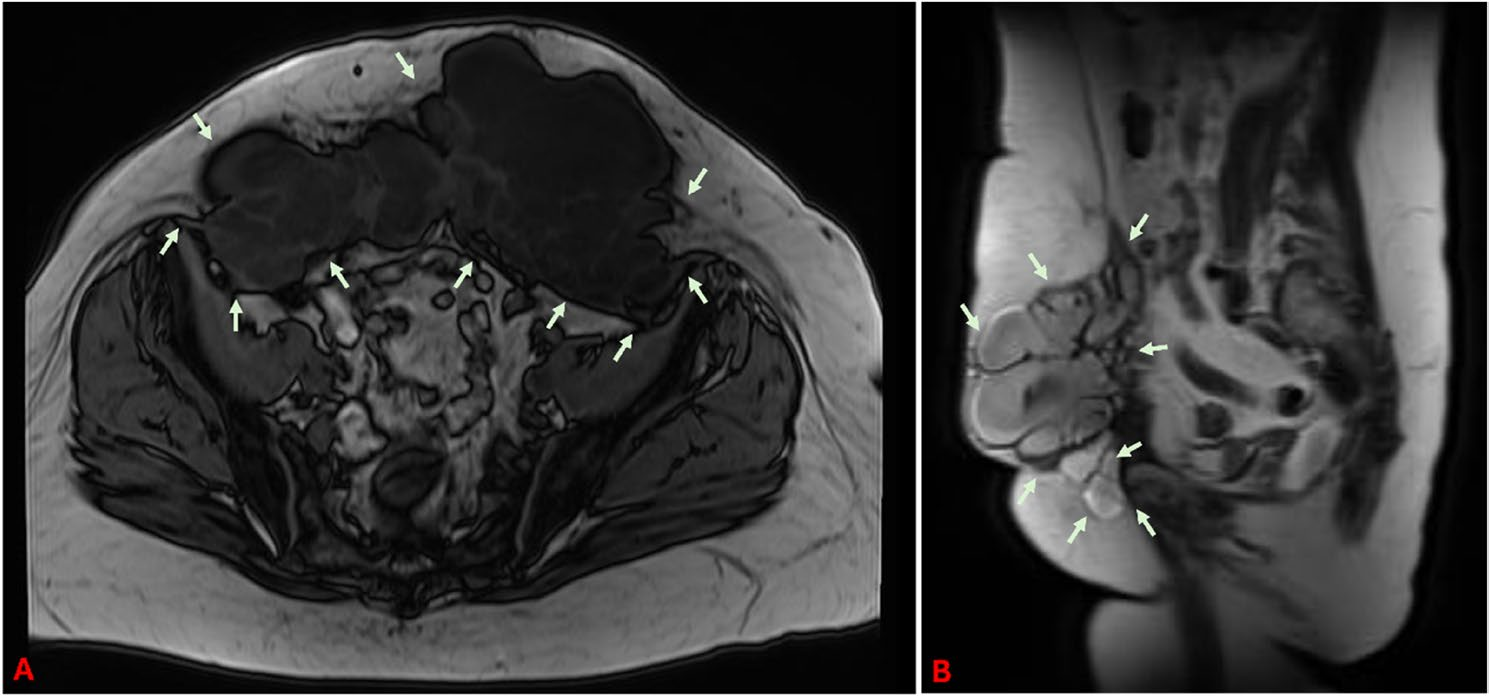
Magnetic resonance imaging (MRI) of the abdominal wall tumor: A: Axial view; B: Sagittal view. We sincerely thank Prof. Dr. Beer, Medical Director of Radiology at Ulm University Hospital, and his team for kindly providing the images. The tumor is marked with arrows
